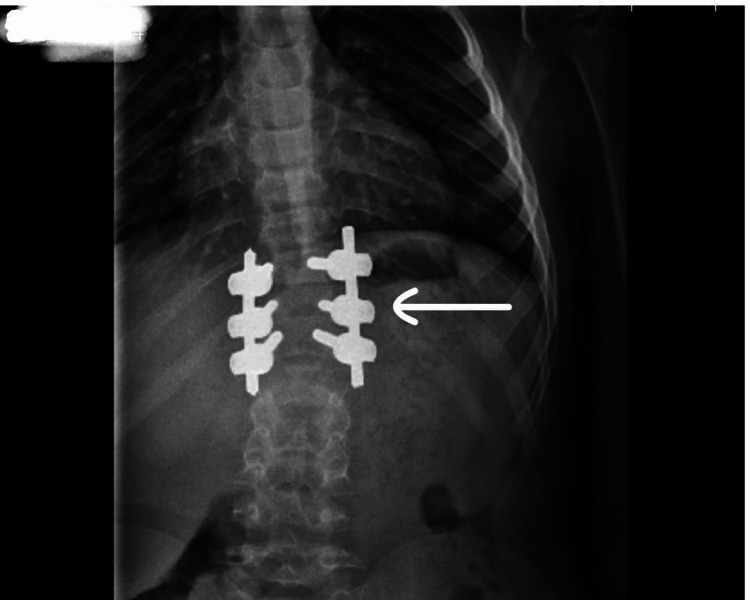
Anteroposterior X-ray view: Postoperative image showing spinal fixation with metal implants in a patient with Morquio syndrome, demonstrating vertebral abnormalities and scoliosis correction.
